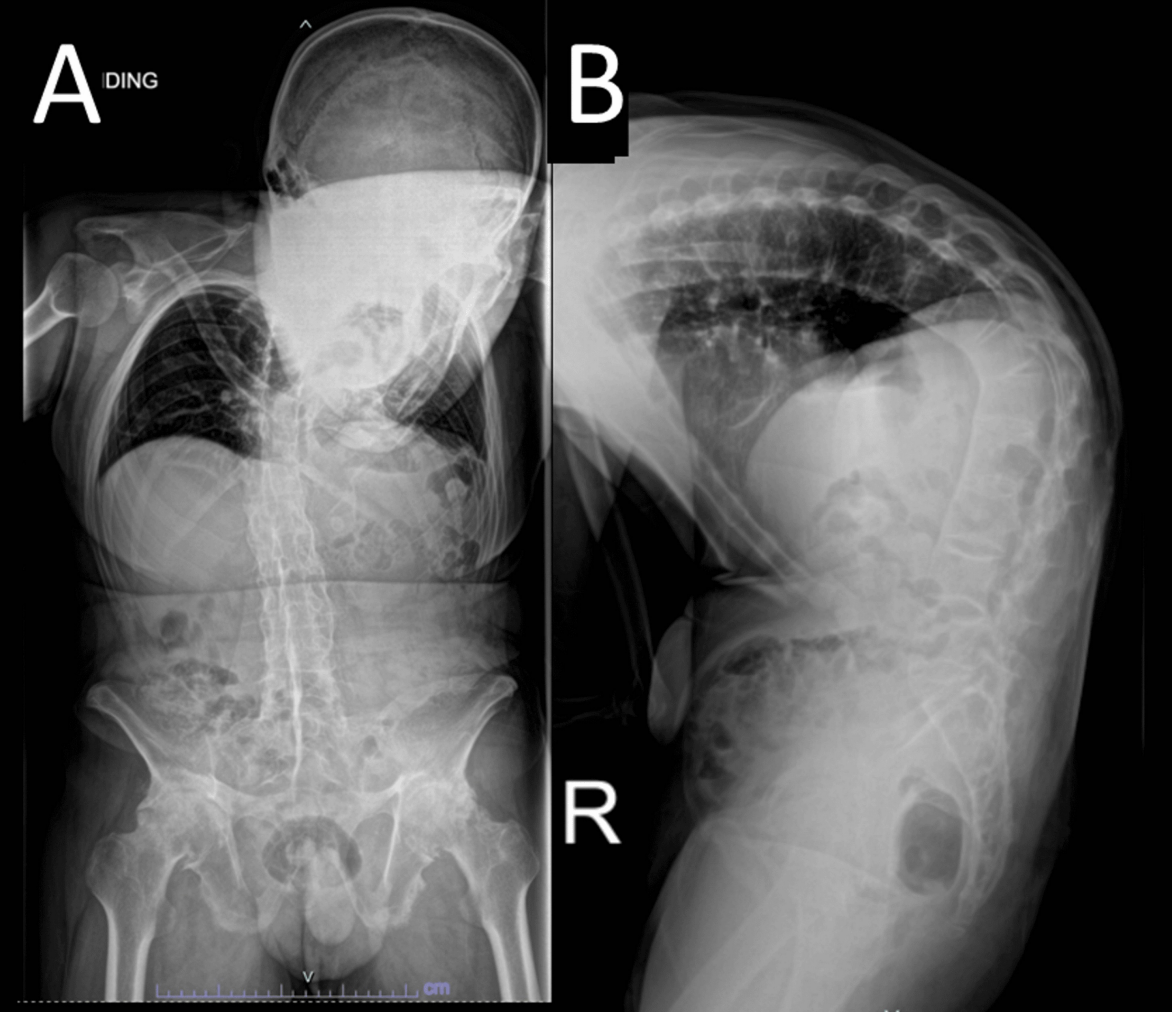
Spinal X-ray before operation Figure 2A: X-ray (anteroposterior view) of the whole spine of the patient; Figure 2B: X-ray (lateral view) of the whole spine of the patient.
